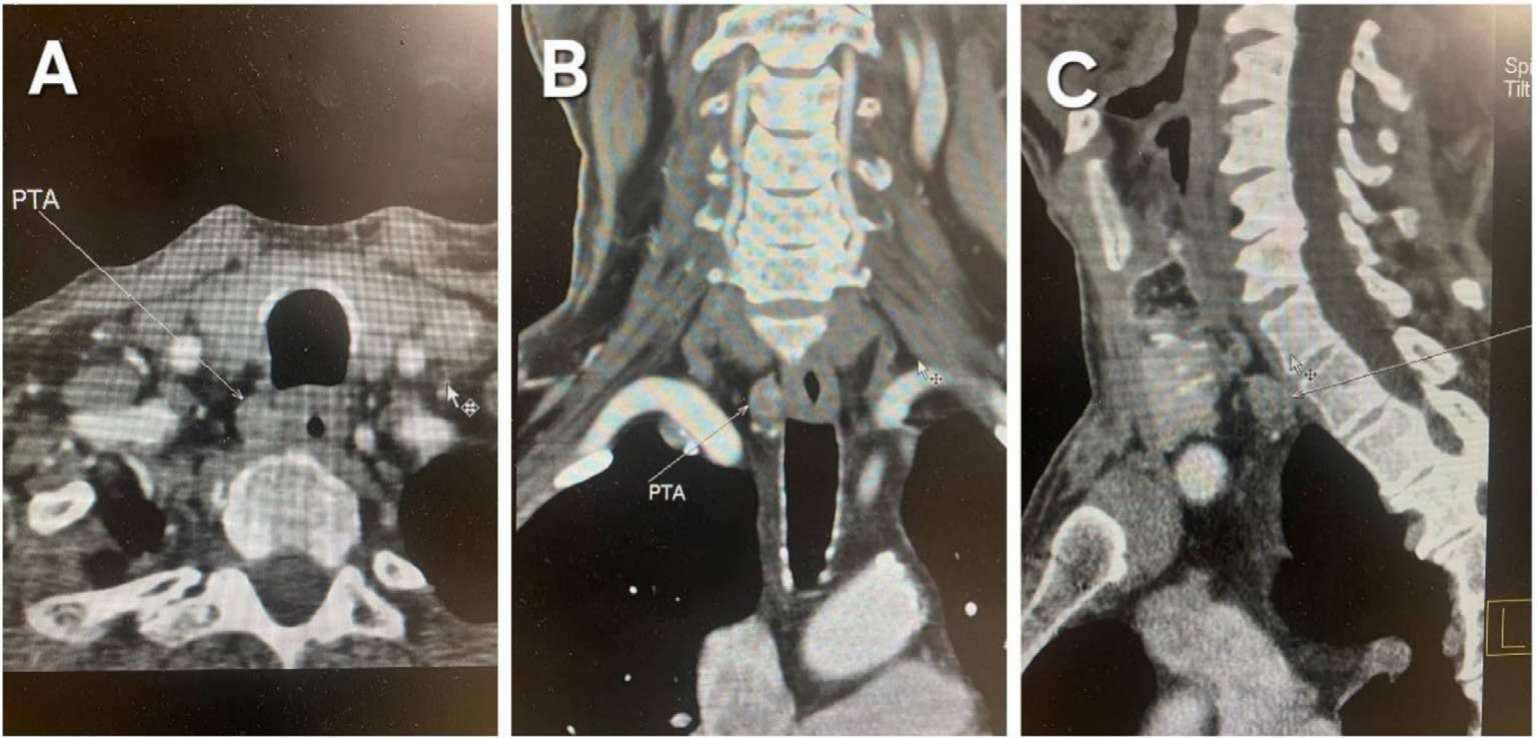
Neck CT scan with contrast study in axial (A), coronal (B), and sagittal (C) views: A 24 × 14 mm hyperenhancing, well‐circumscribed, ovoid nodule in the inferior right retrothyroid region of the right paratracheal space, consistent with parathyroid adenoma.
